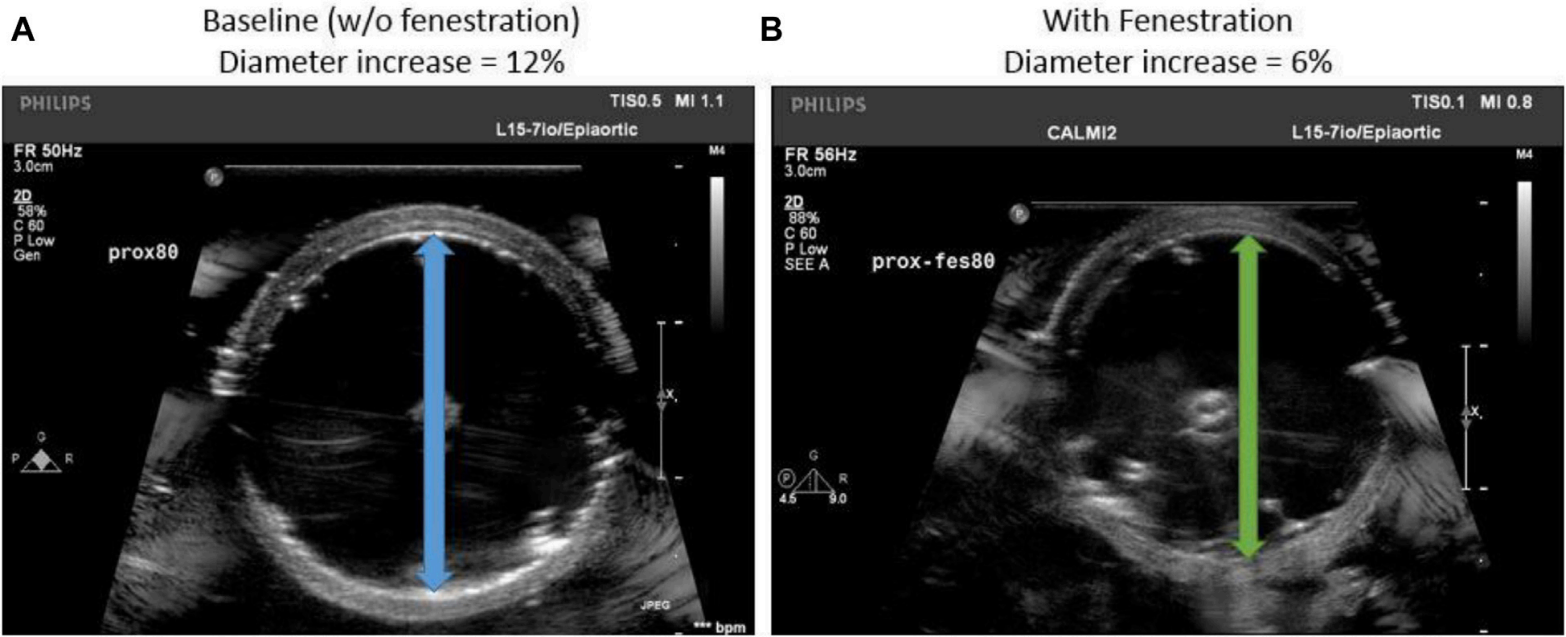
(A) Transverse ultrasound image of the baseline aorta suspended in the benchtop setup (B) An aorta flap fenestrated with optimal slit fenestration pattern is re-apposed using a balloon.
